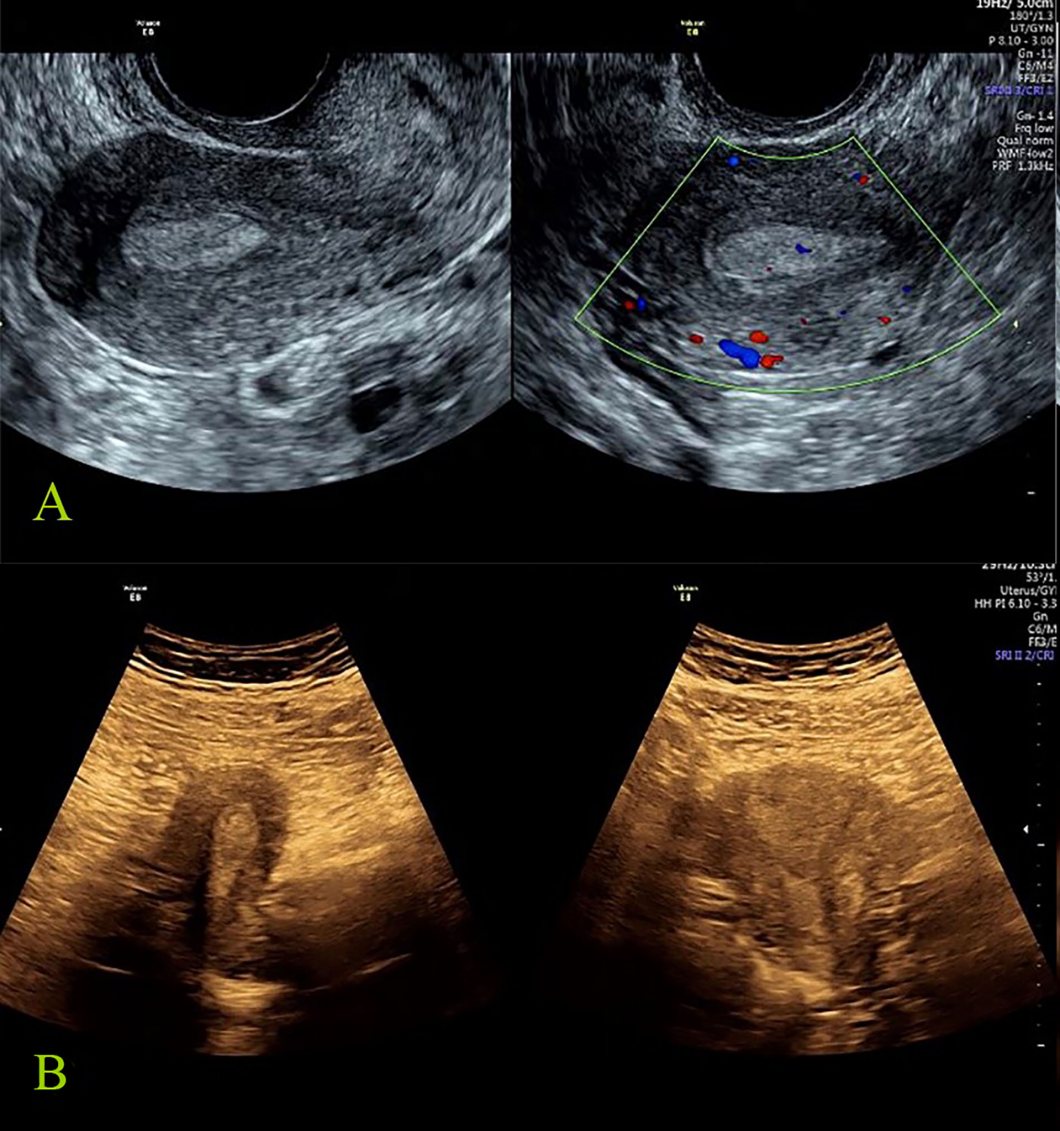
Benign reference case: female, 27 years old, abnormal menstruation for more than 2 months. All the seven tumor biomarkers were normal, the postoperative pathology was endometrial polyp, and the total score of IETA ultrasound characteristics simple scoring method was: 3 points [endometrial thickness 10 mm: 0 point; heterogeneous without cysts: 1 point; regular endometrial–myometrial junction: 0 point; having “Bright edge” sign: 0 point; color score: minimal color/sparse vascularity: 2 point; single vessel (without branching): 0 point]. (A) The grayscale and color Doppler ultrasound images of endometrial polyp (transvaginal scan). (B) The grayscale images of endometrial polyp (transabdominal scan).
